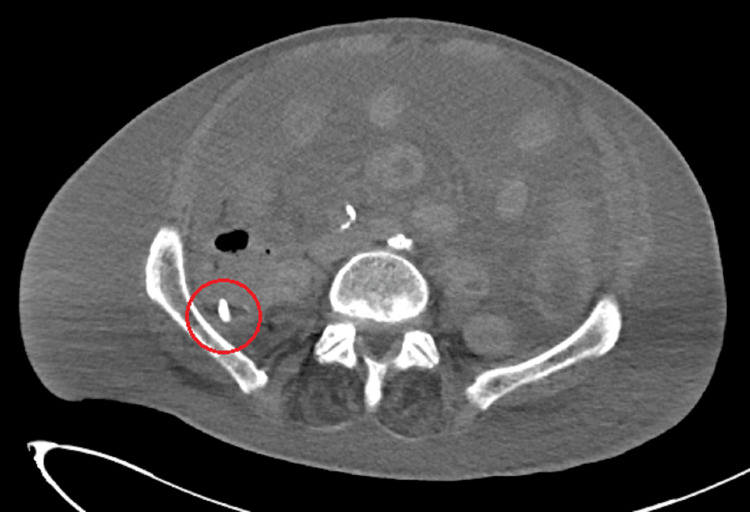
Axial view CT abdomen/pelvis showing ascites and a hyperdensity in the right pelvis concerning for a foreign body.
